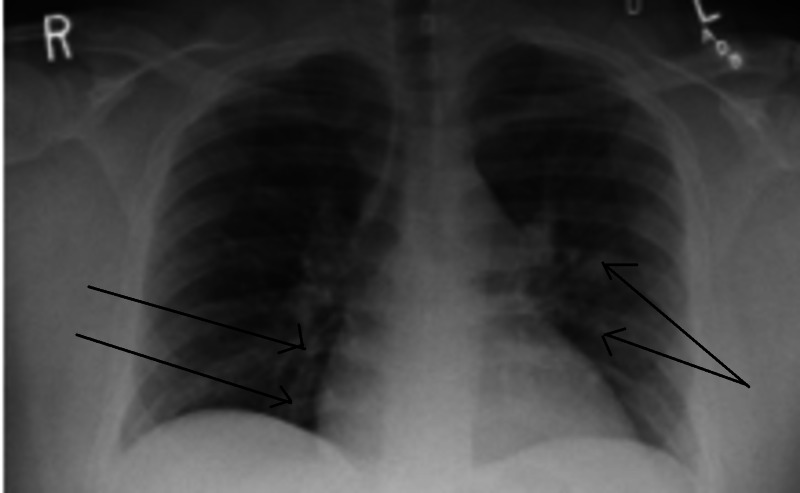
Chest X-ray Patchy opacities in bilateral lung fields
